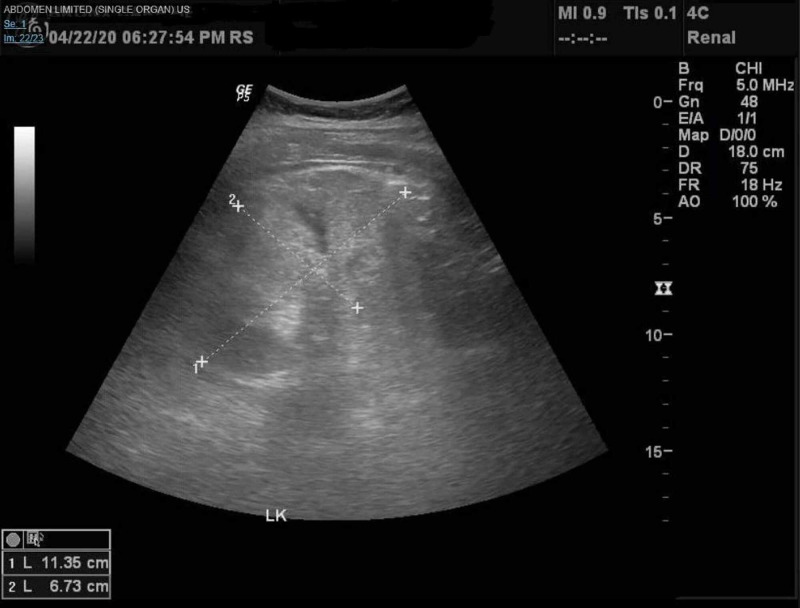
Renal Ultrasound - Left kidney
